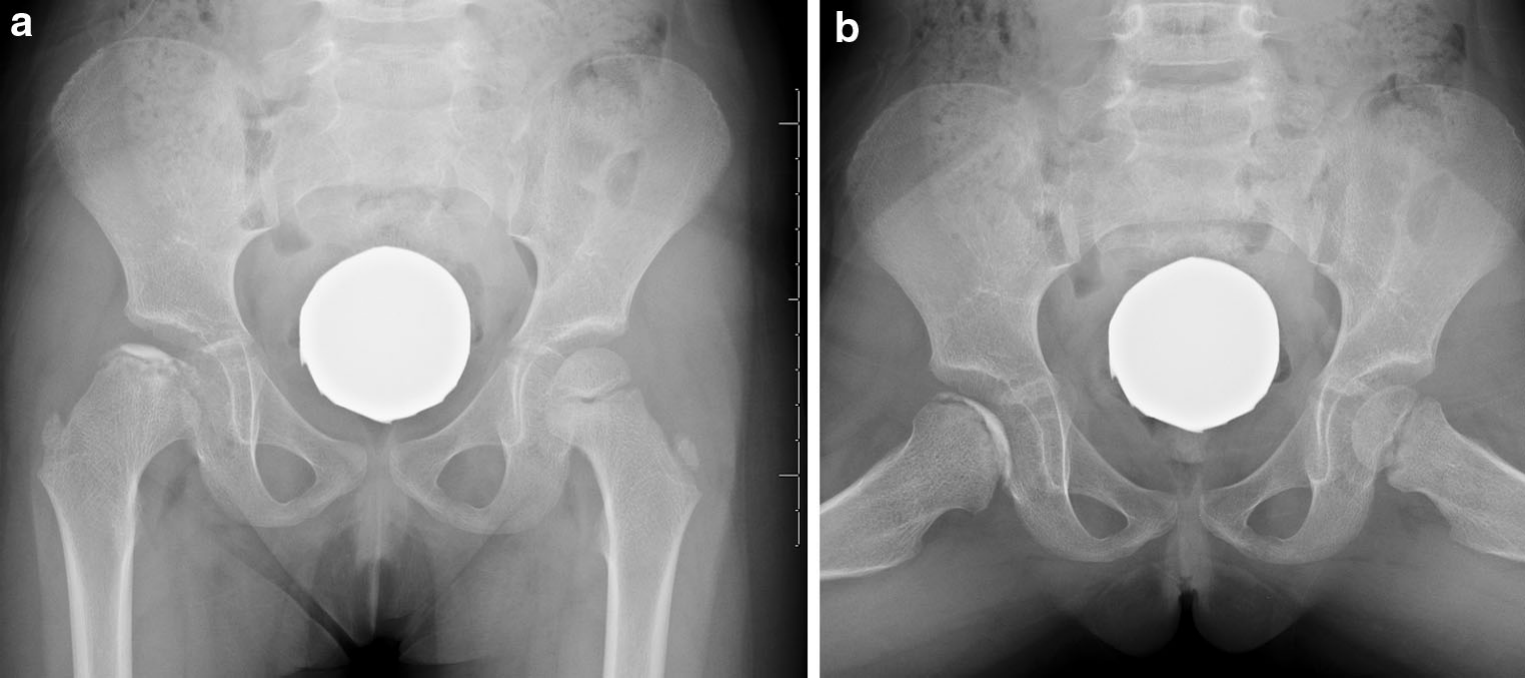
AP and frog lateral radiographs prototypical of the presentation of female Perthes disease, with femoral head fragmentation and loss of height of the lateral pillar
